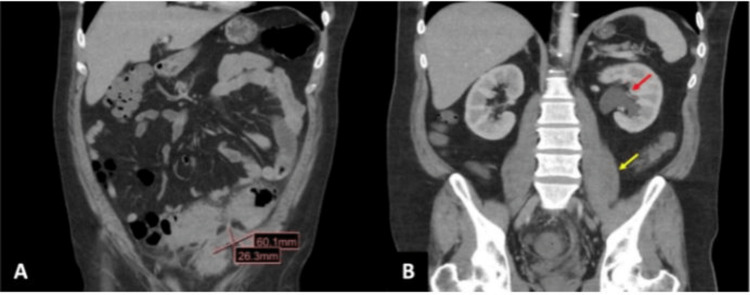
(A) Sigmoid diverticulitis with perisigmoid abscess measuring 6 cm × 2.6 cm. (B) Left-sided hydroureter (yellow arrow) and hydronephrosis (red arrow) noted on the computed tomography scan of the abdomen with contrast.
